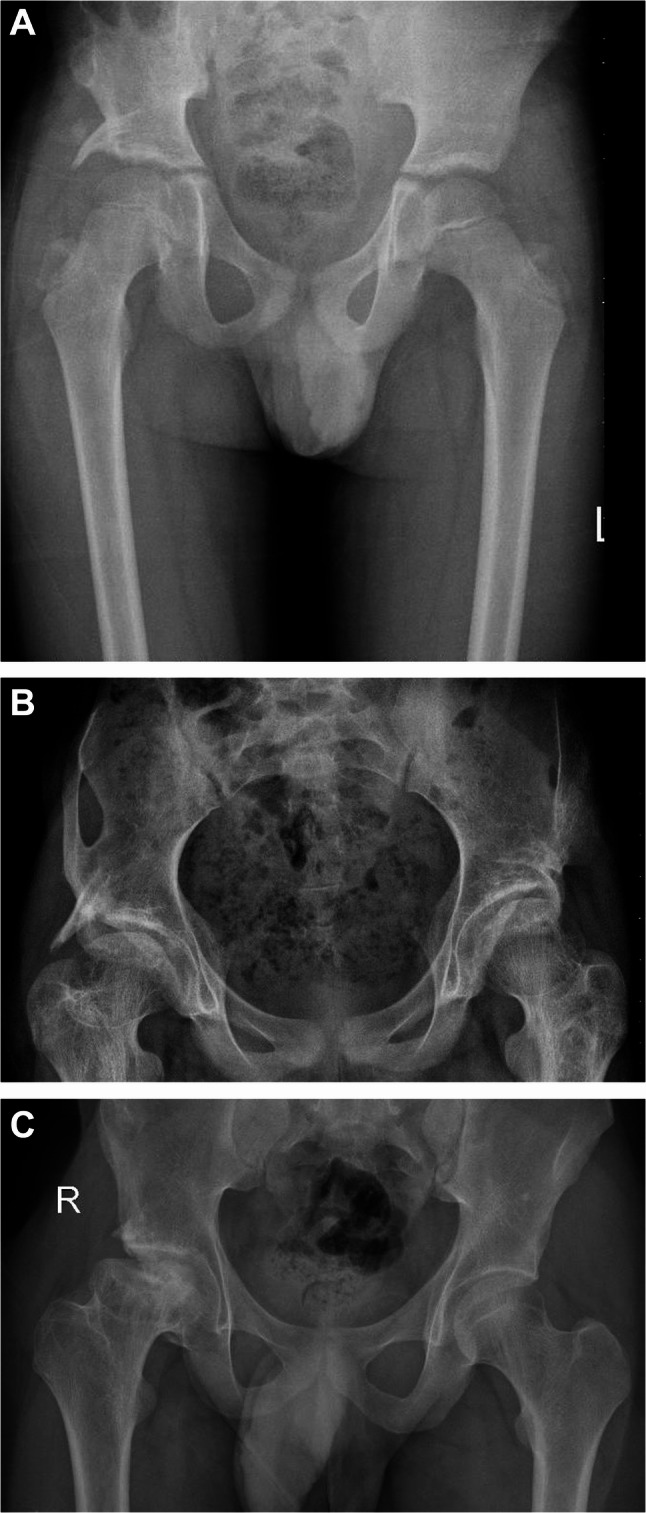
A A male patient, diagnosed with an ONFH Lateral Pillar grade B at the age of 6-y. Radiograph done at the age of 11-y, 59-m following SA, demonstrating a grade- 1 Stulberg of the right hip. B A female patientdiagnosed with bilateral ONFH lateral pillar grade B at the age 8-y. Radiograph done at the age of 11.9-y, 48-m following SA, demonstrating a grade- 3 Stulberg of the right hip and grade-2 of the left hip. C A male patient, diagnosed with ONFH Lateral Pillar grade B at the age of 13-y. Radiograph done at the age of 17-y, 60-m following SA, demonstrating grade-5 Stulberg of the right hip
